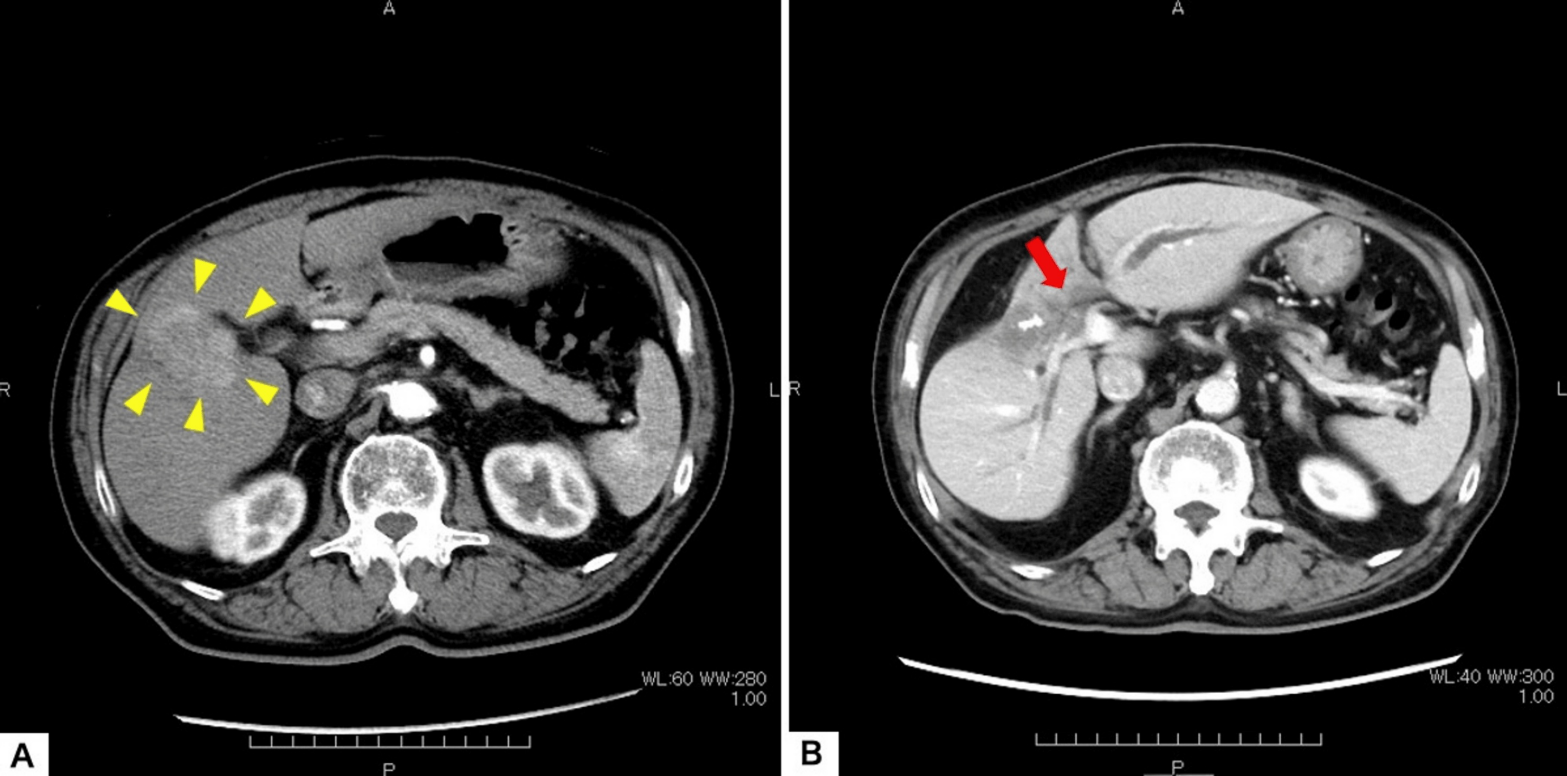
Radiographic evolution of radiation-induced biliary stenosis (RIBS). (A) Pre-treatment contrast-enhanced computed tomography (CT) (three months prior to carbon-ion radiotherapy (C-ion RT)) showing the viable hepatocellular carcinoma (HCC) in Segment 4/5 (arrowheads). (B) CT at the onset of jaundice (2.5 years post-C-ion RT) showing marked atrophy of S5 (arrow) and significant upstream biliary dilation (arrow).
